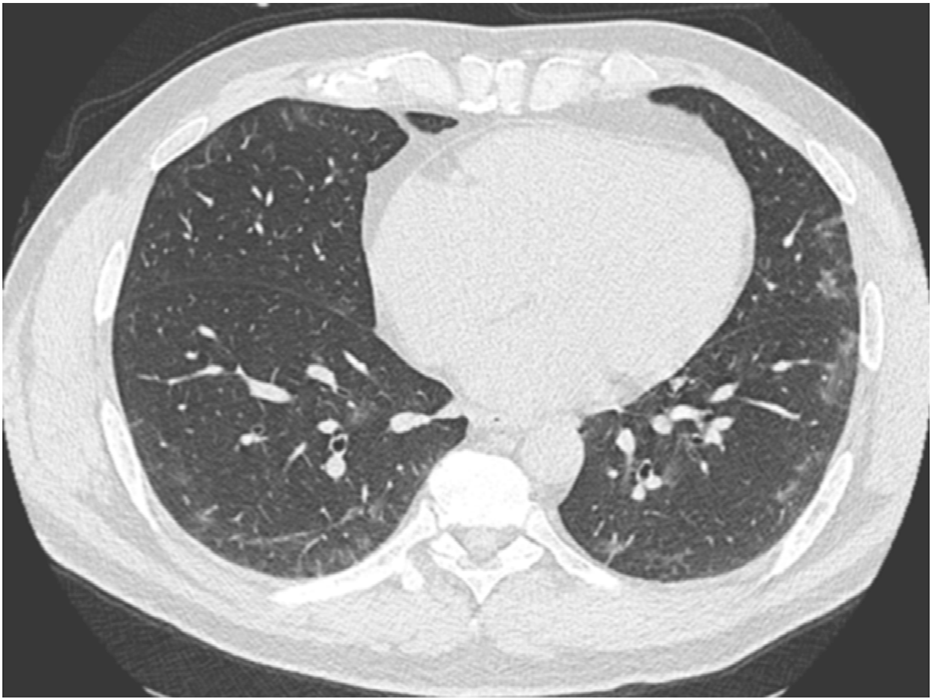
Asymptomatic diver with a chest CT scan performed 1 month after a positive PCR test. A characteristic appearance of very mild COVID-19-related lung involvement with limited bilateral ground glass opacities can be noted.
